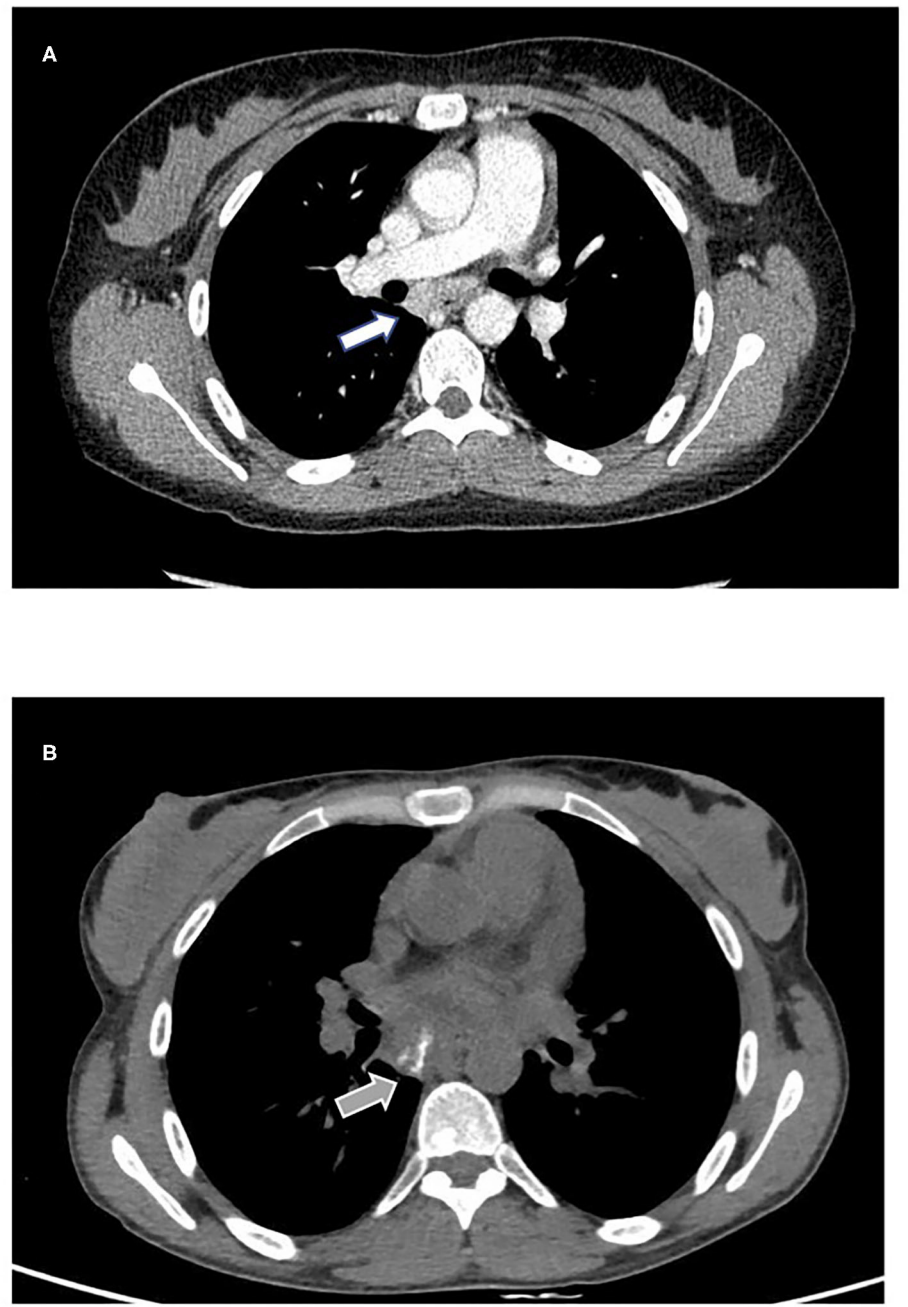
Mediastinal involvement. (A) Contrast enhancing subcarinal lymphadenopathy detected with CT scan performed in 2019 (white arrow). (B) One year follow up shows increased volume and intranodal calcification (gray arrow). No parenchymal abnormalities were found.
